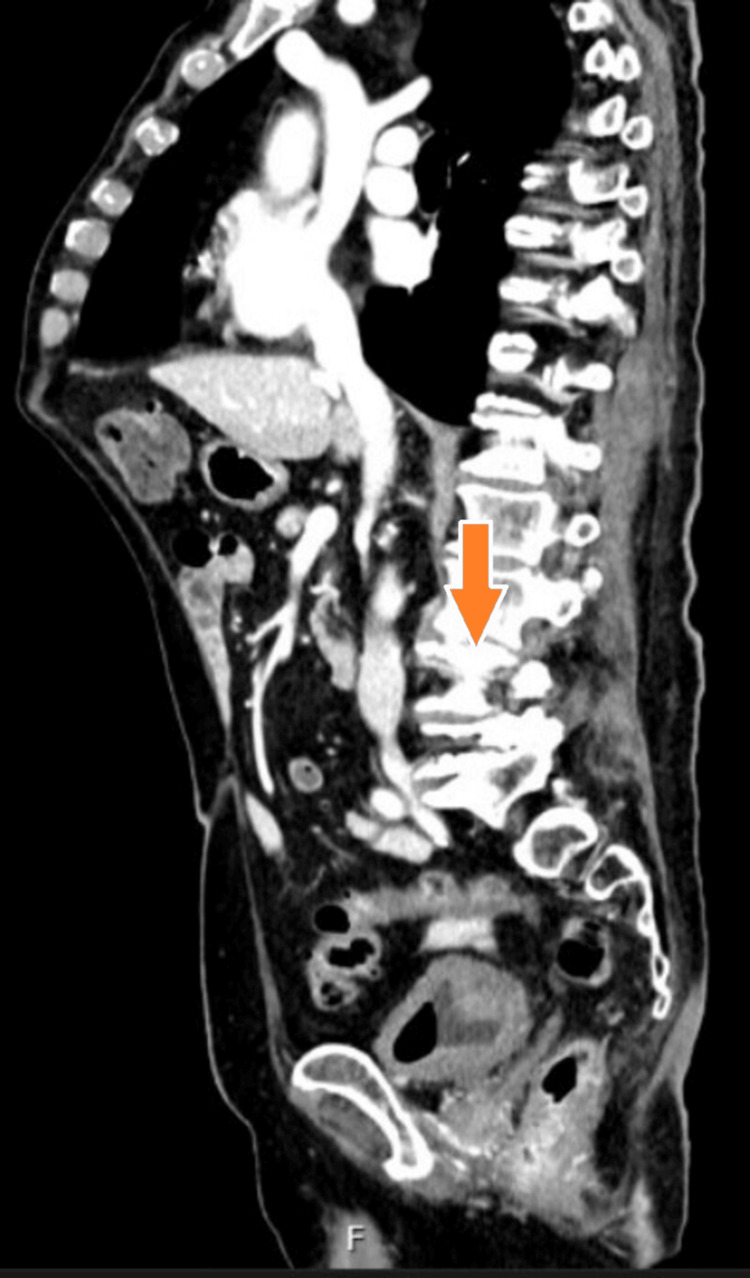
Sagittal CT scan of the lumbar spine demonstrating destructive changes at the L2/L3 intervertebral space, consistent with spondylodiscitis. The orange arrow highlights the area of vertebral involvement.
